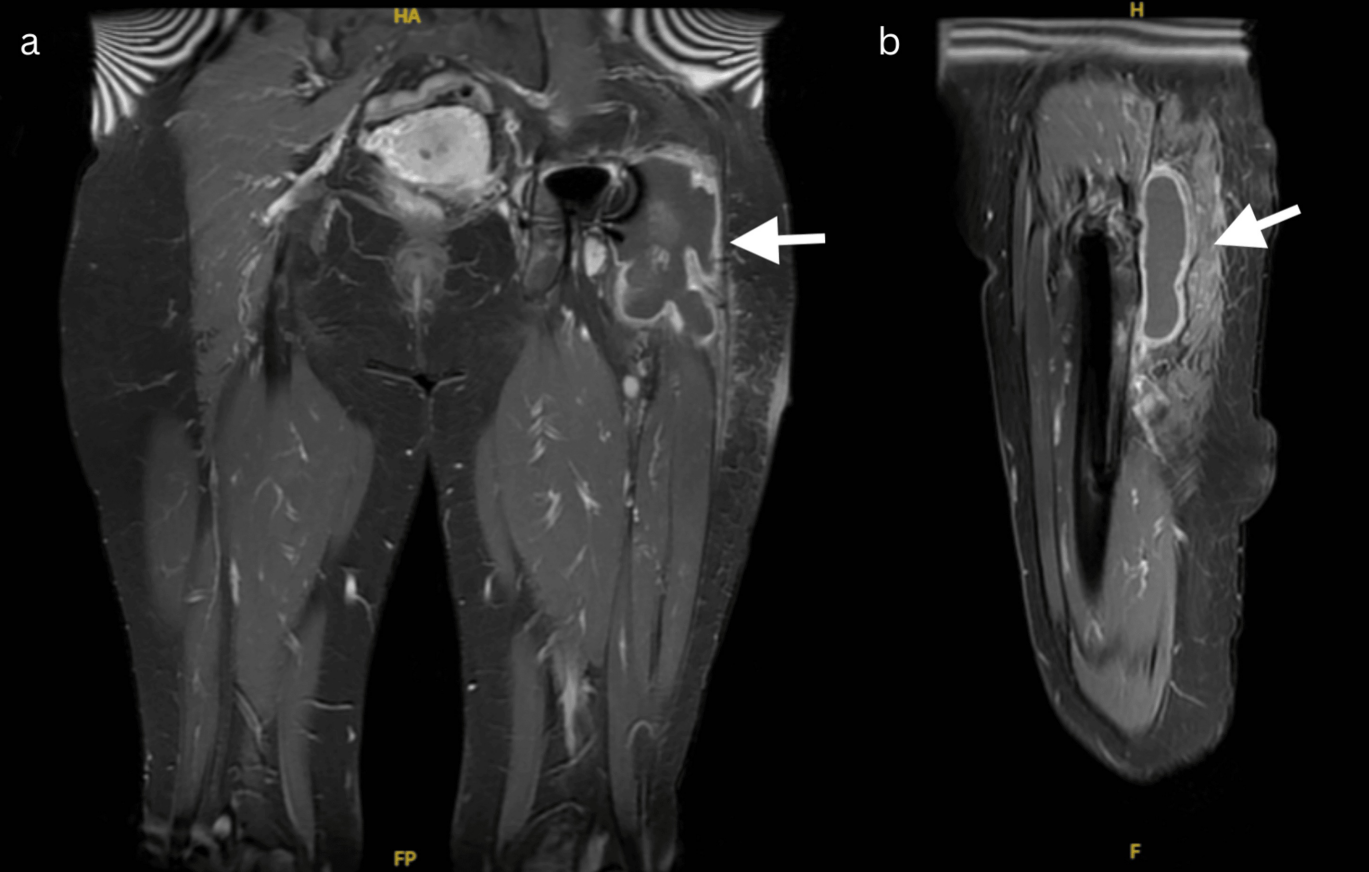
Magnetic resonance imaging showed a large complex fluid collection (3.9 cm × 7 cm × 9.6 cm) with a low signal on T1 in coronal (a) and sagittal view (b)
